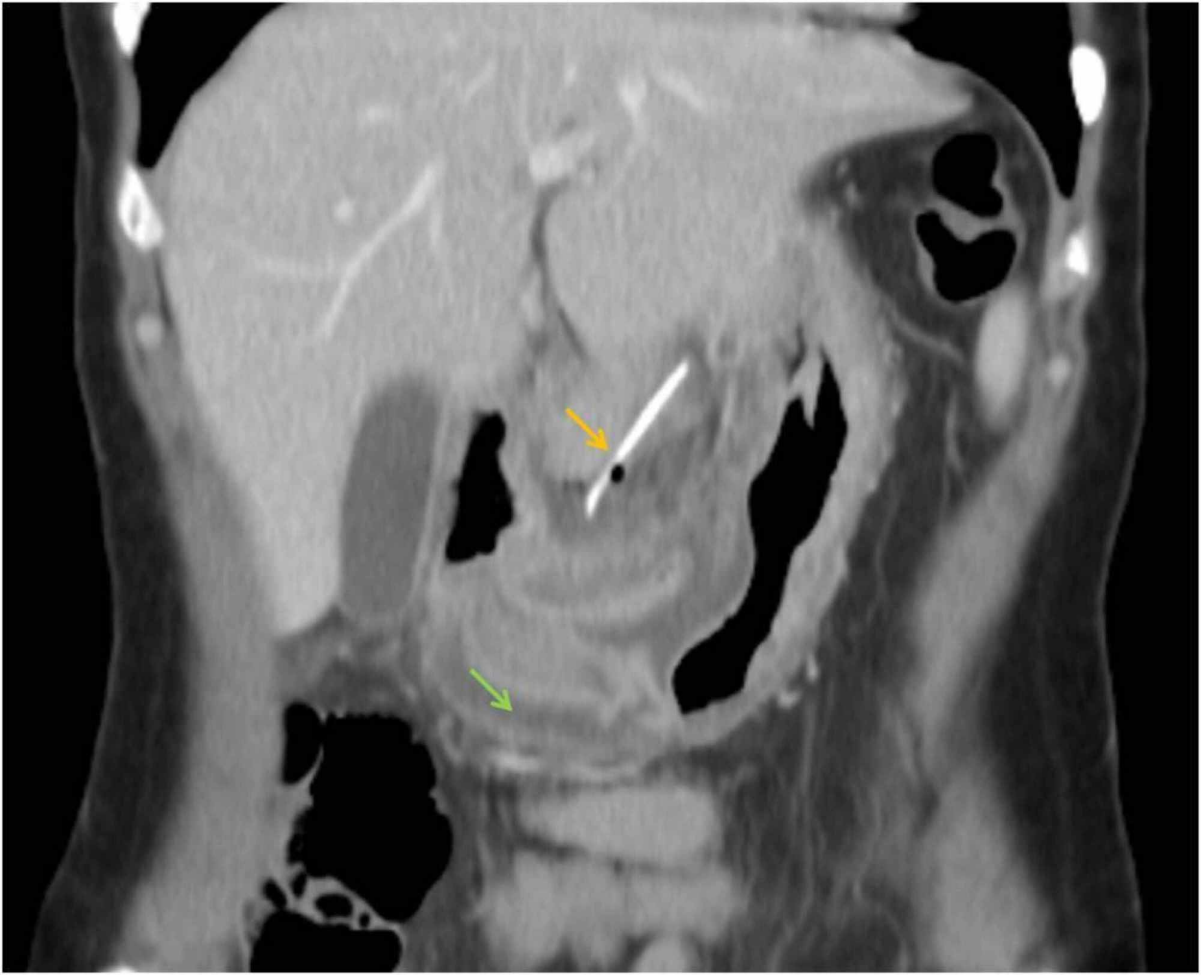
Abdominal CT: coronal reconstruction The yellow arrow points a linear high-density structure proven to be a fish bone migrated to the lesser omentum. The green arrow shows thickened gastric walls due to local inflammation and peritonitis caused by antral perforation. Minimal amount of extraluminal and free fluid is seen near the fish bone due to a contained inflammatory process.
